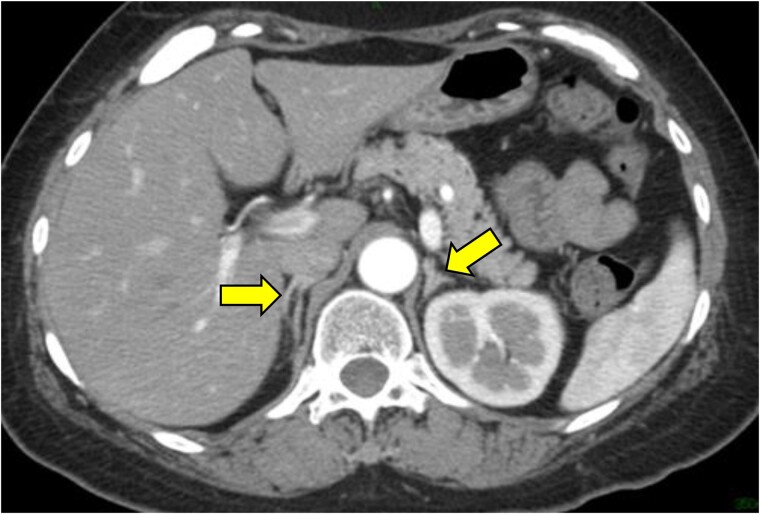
Abdominal computed tomography (CT) imaging. No apparent tumor was observed from the CT imaging. The yellow arrows indicate adrenal glands.
